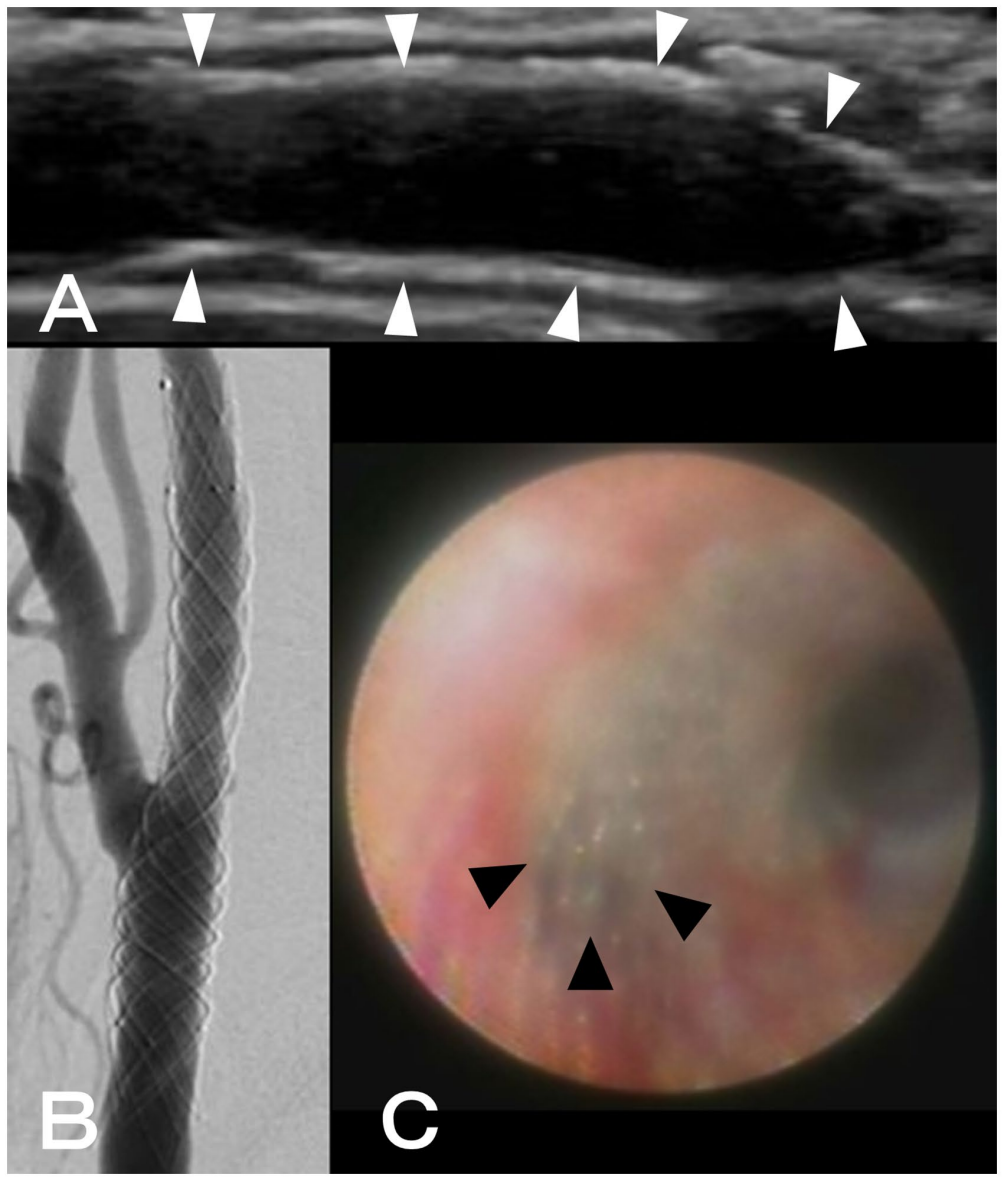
(A) Using ultrasonography, neointimal formation in CASPER Rx could not be detected (white arrowheads: CASPER Rx). (B) A radiolucent gap between the stent and artery lumen was discovered during right common carotid angiography. (C) Using angioscopy, thin neointimal development was seen throughout, except for the external carotid artery orifice (black arrowheads: the orifice of the external carotid artery).
